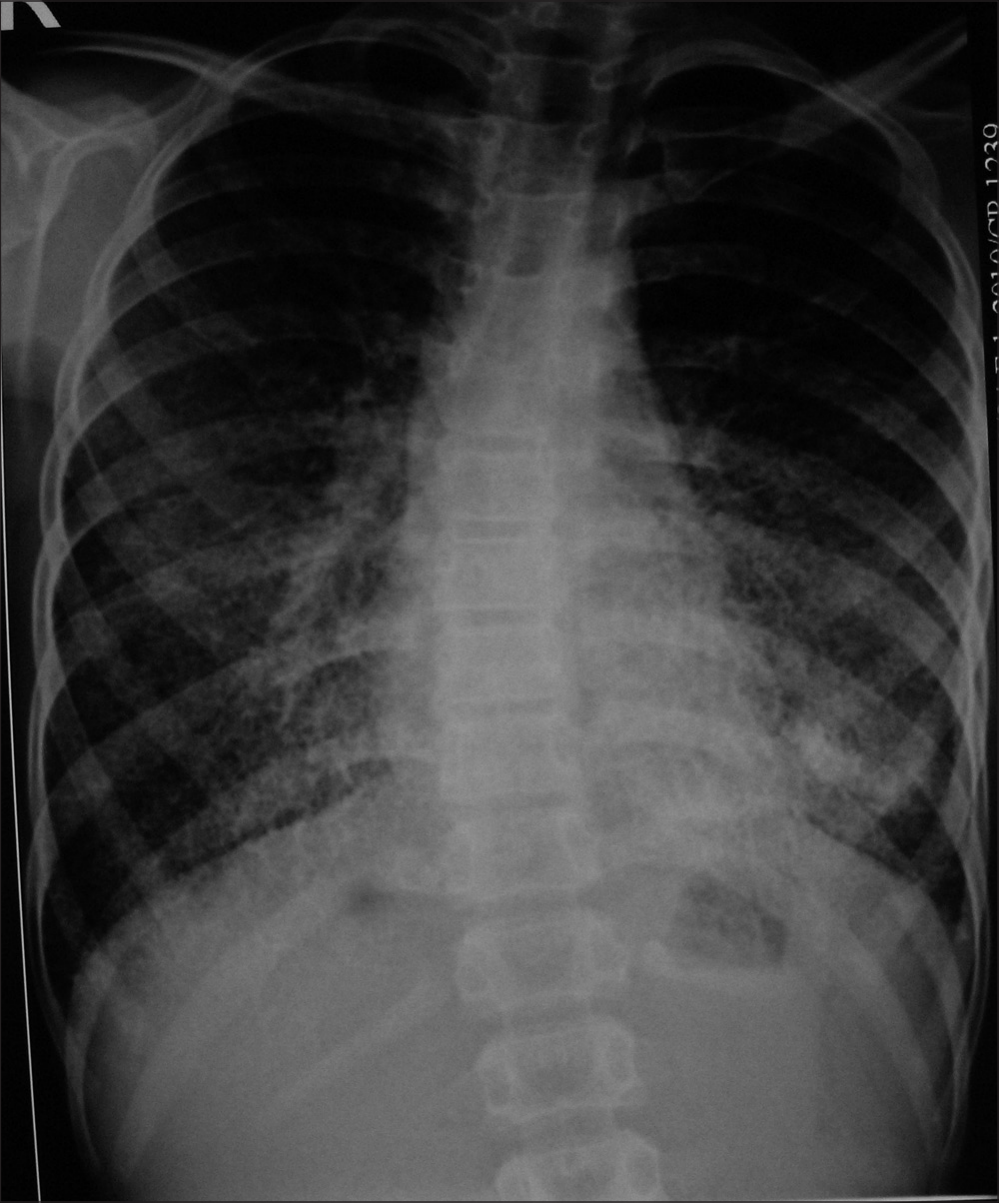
Chest X-ray showing bilateral diffuse high density micronodular opacities more toward the mid and lower zones
